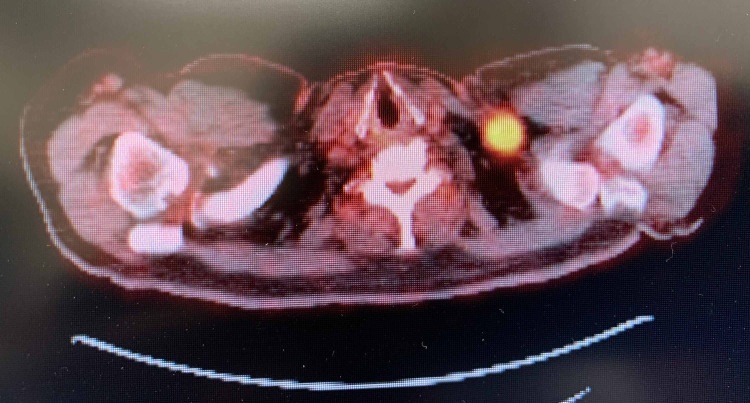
PET scan Enlarged, hypermetabolic lateral left supraclavicular lymph node with maximum SUV of 6.8 SUV: standardized uptake value; PET: positron emission tomography
